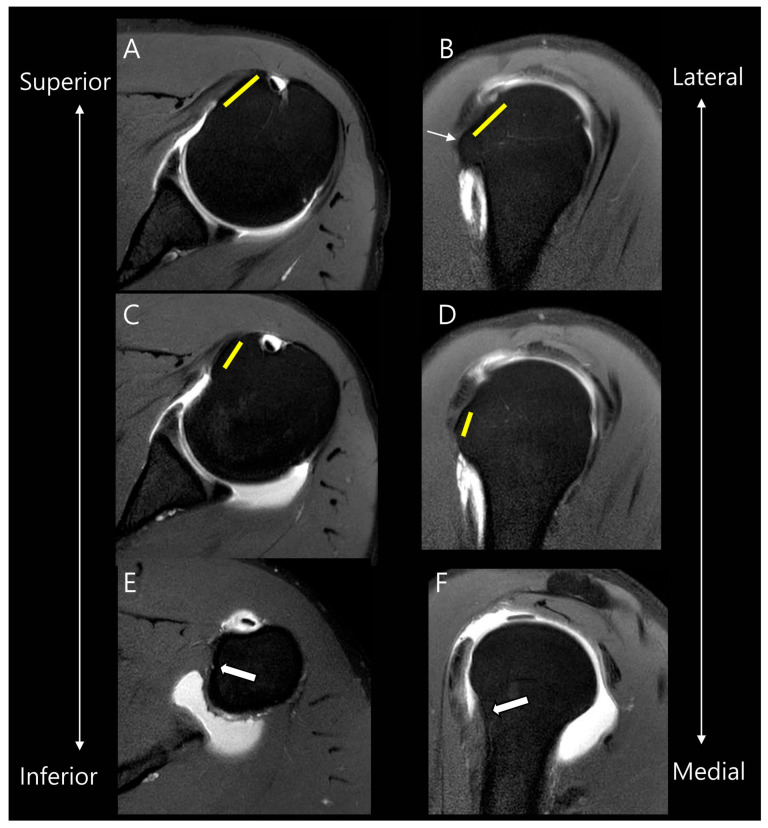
Anatomy of facets of the subscapularis tendon on MR images. On oblique axial and oblique sagittal MR images, the first facet (yellow lines in (A,B)) is located more superiorly and laterally compared to the second facet (yellow lines in (C,D)). They are separated by a bony ridge (thin arrow in (B)). The following third and fourth facets represent the large muscular insertion in the inferior attachment (thick arrows in (E,F)).
